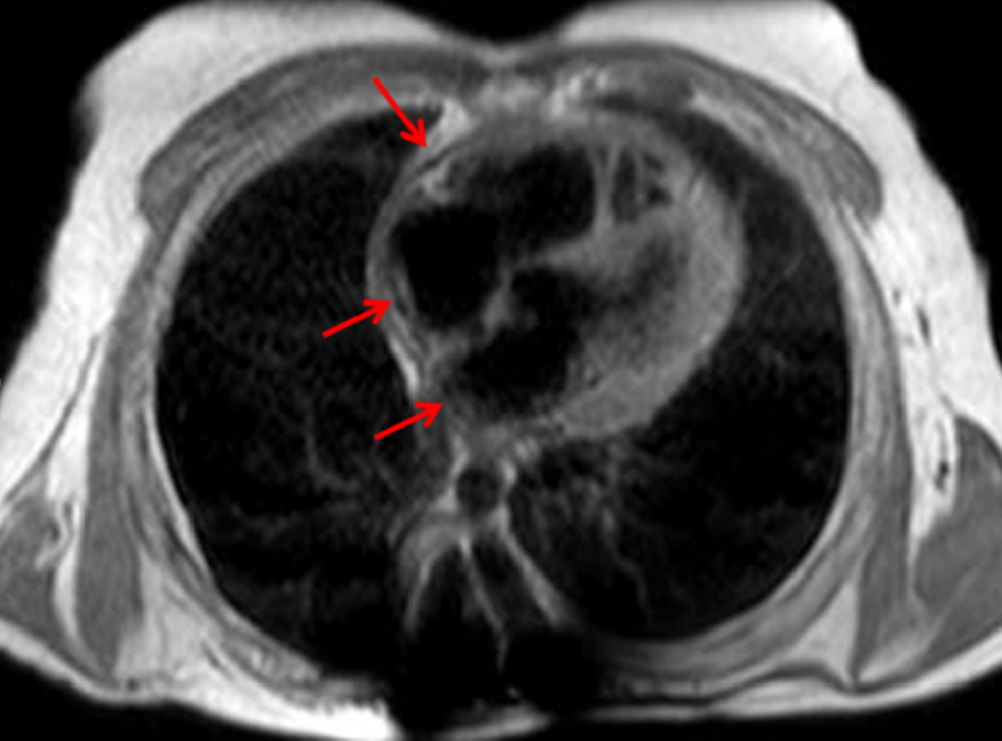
MRI axial “black-blood” sequence. Hypointense linear images (arrows) correspond to the calcifications shown in CT.
